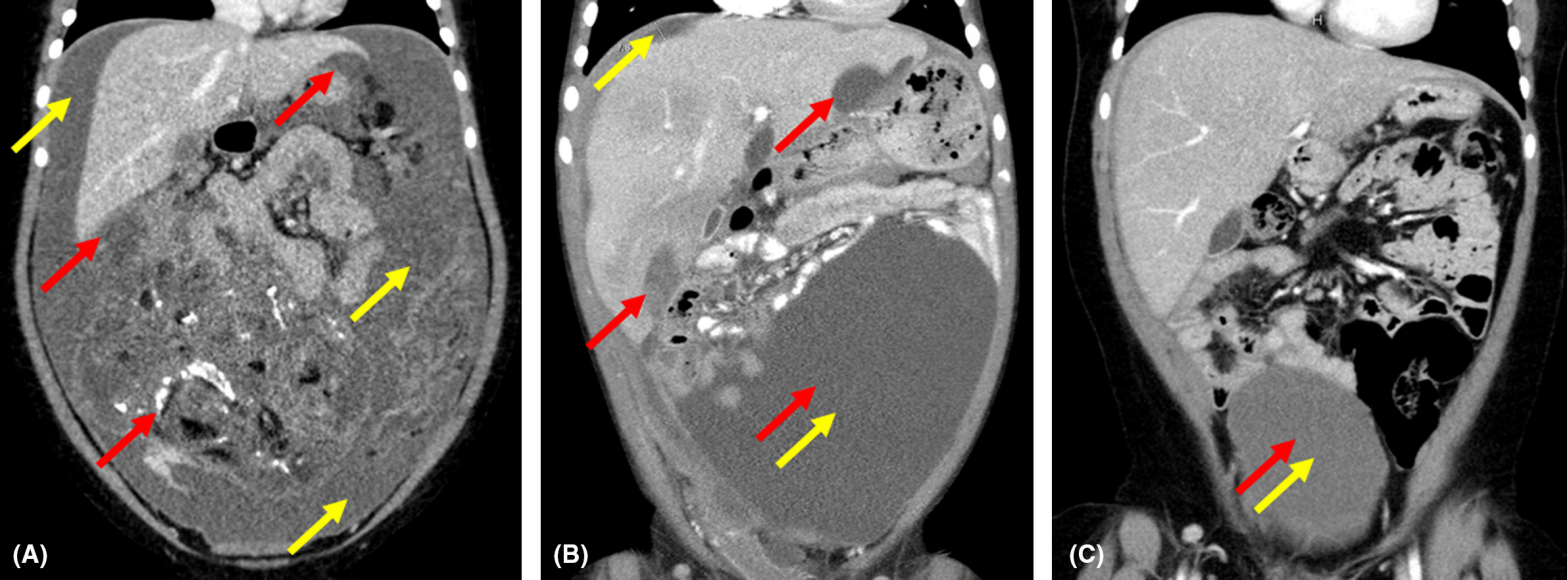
Side‐by‐side comparison of coronal CT imaging on diagnosis notable for significant ascites and large mass (A), relapse with recurrent ascites (B), and after 5 years on crizotinib therapy without malignant ascites and persistent necrotic mass (C). Red arrows represent areas of tumor, and yellow arrows represent areas of malignant ascites. When both red and yellow arrows are close together, this area represents known tumor that is obscured by malignant ascites in the pelvis.
